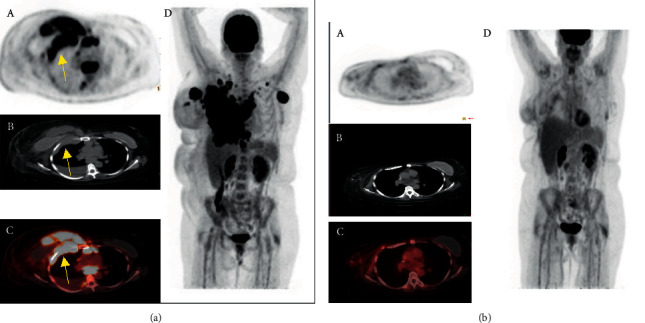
(a) Baseline: (A) axial PET, (B) CT, and (C) fused images from an FDG-PET/CT scan performed at baseline demonstrate a large, infiltrative mass in the right chest wall measuring approximately 13 x 10 x 20 cm. The mass spans the subcutaneous, muscular, and chest wall compartments with extension into the pleural/extrapleural compartments (arrows). (D) A maximum intensity projection (MIP) image shows the large extent of disease. (b) Post treatment: (A) Axial PET after treatment with 6 cycles of CHP/BV, Surgery and XRT, (B) CT, and (C) fused images with stable postsurgical changes showing (d) overall significant positive response with slight interval increase in interstitial opacity in the central right breast with low-grade FDG uptake thought to be related to inflammation post radiation.
